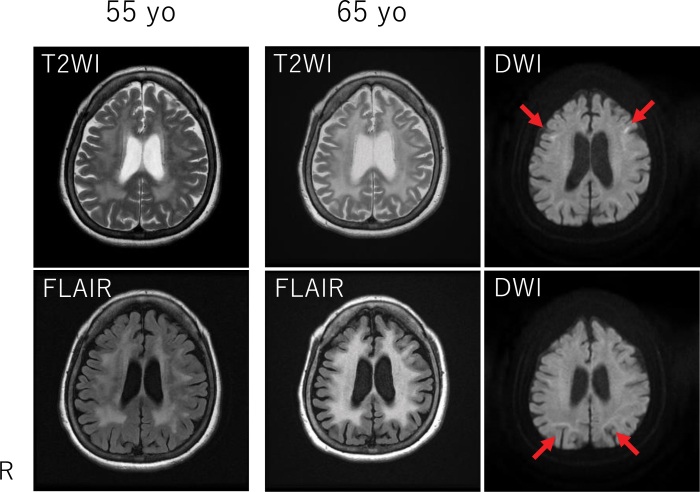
Brain magnetic resonance imaging scans of the patient obtained at the ages of 52 and 65 T2-weighted imaging (T2WI) and fluid-attenuated inversion recovery (FLAIR) showed progressive diffuse confluent white matter changes. Diffusion-weighted imaging (DWI) shows subcortical hyperintense signals in the frontal and parietal lobes (arrows).
